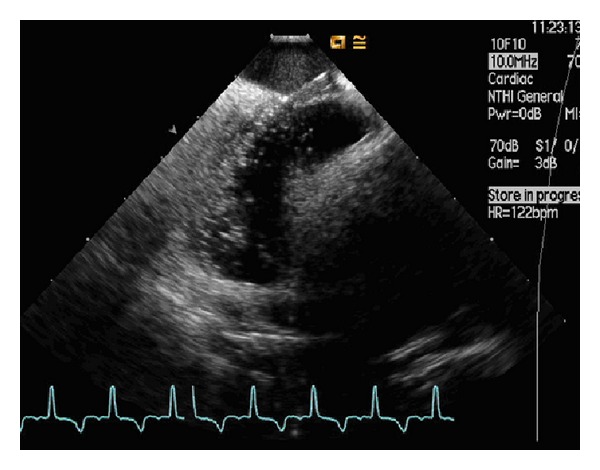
Agitated saline bubble study during intracardiac echocardiography. Agitated saline bubbles are shown into the LA confirming successful septal puncture. Subject's heart rate and rhythm are unchanged, indicating good tolerance to this manoeuvre.
